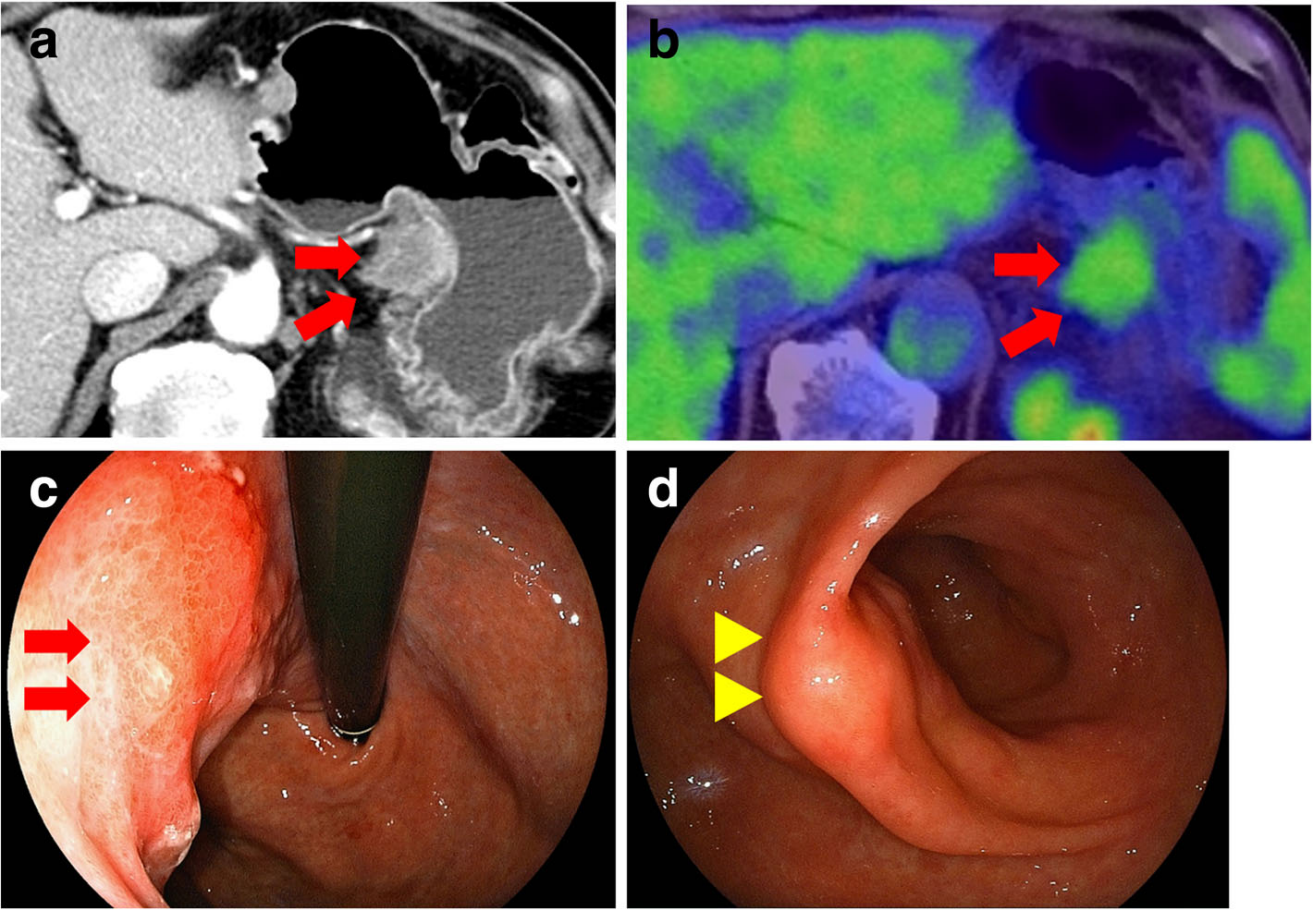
Imaging diagnosis of recurrence. a MDCT showed a 30-mm nodule at the lesser curvature of the stomach. b Positron emission tomography (PET)/CT showed fluorodeoxyglucose (FDG) uptake in the nodule; SUVmax was 3.5. c Upper gastrointestinal endoscopy revealed mucosal irregularity in the posterior wall of the lesser curvature of the gastric body (arrow). d Upper gastrointestinal endoscopy revealed submucosal tumor in the anterior wall of the gastric antrum (arrowhead)
